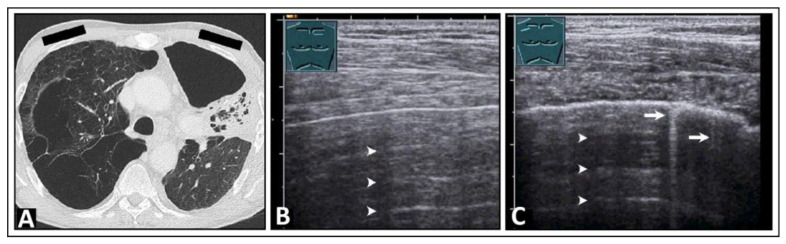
A patient with shortness of breath and known bullous emphysema in the context of chronic obstructive pulmonary disease following volume-reduction surgery due to recurrent pneumothoraxes. (A) On computed tomography, the bullous lung emphysema is shown with a large right apicoventral bulla; the black rectangles indicate the positions of the ultrasound probe. (B) On lung ultrasound, in the left upper lobe, a sharp standing reflective line with A-lines (arrowheads) without comet-tail artifacts is striking. A pneumothorax cannot be ruled out sonographically. (C) On lung ultrasound, the right upper field shows a somewhat rough pleura with comet-tail artifacts (arrows) and A-lines (arrowheads).
